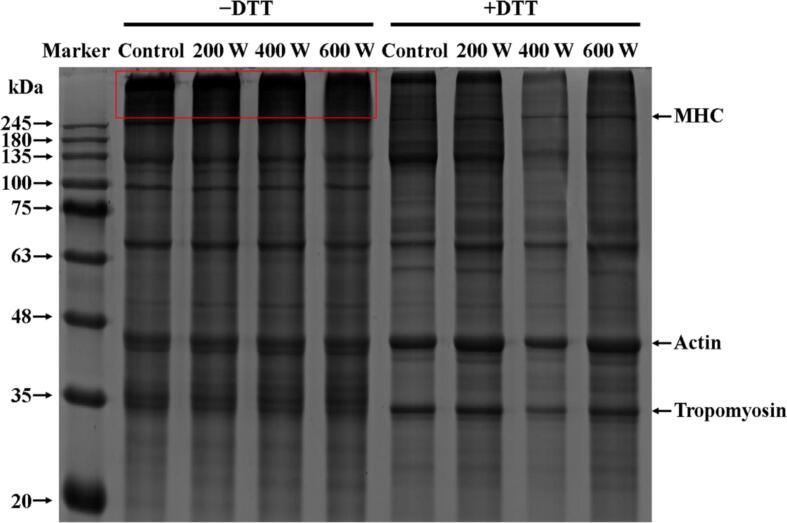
Effect of different ultrasound power on the protein composition of *N. taihuensis* MP.
